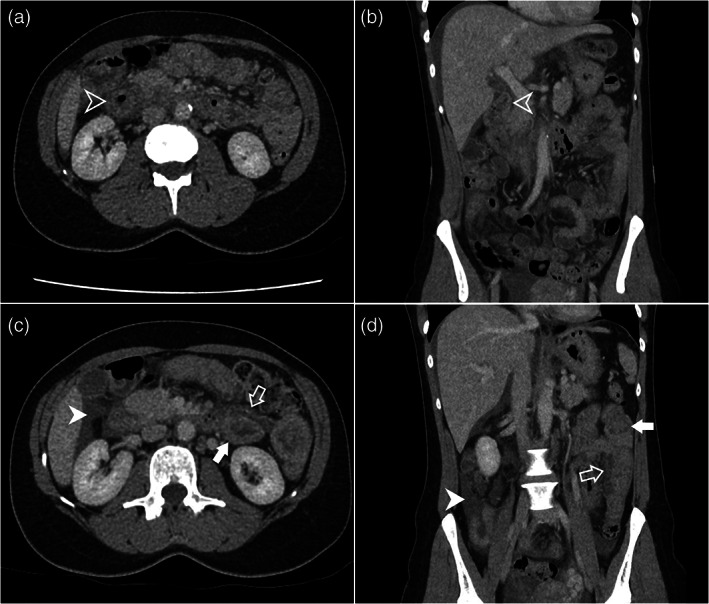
(a) Axial and (b) coronal computed tomography show duodenal thickening with submucosal oedema (empty arrowhead) with periduodenal oedema. (c) Axial and (d) coronal computed tomography, in the same patient, show small bowel wall thickening with submucosal oedema (solid arrow), and adjacent mesenteric oedema (empty arrow), as well as ascites (solid arrowhead).
